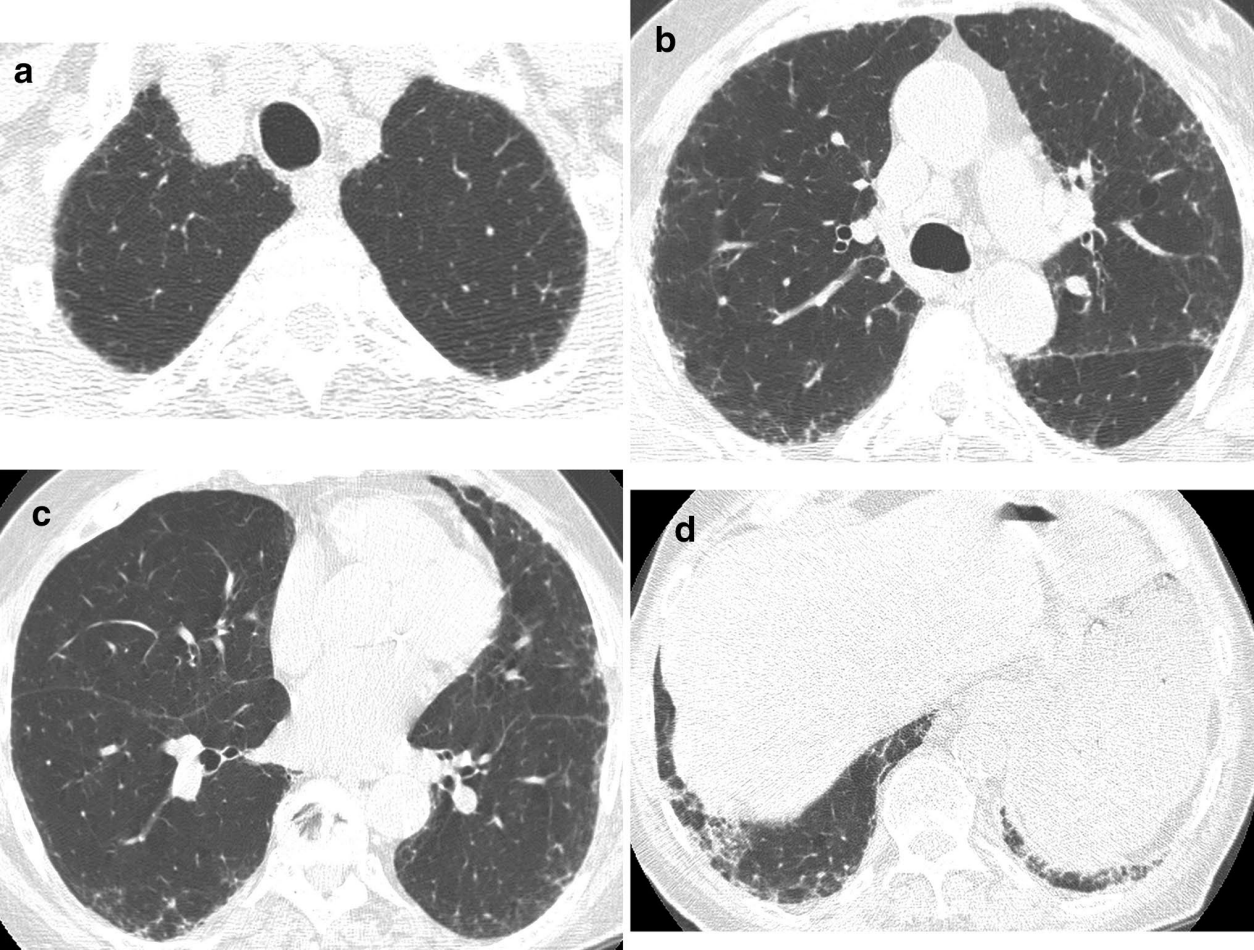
Axial (a–d) HRCT images show peripheral and basilar predominant pulmonary fibrosis characterized by reticulation, traction bronchiectasis, and traction bronchiolectasis but no honeycombing, consistent with a possible UIP pattern
